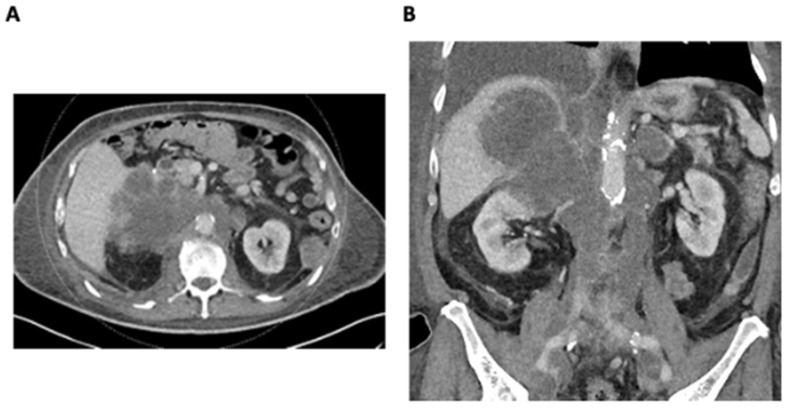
(A) Axial CT demonstrating a large infiltrative right adrenal metastasis and retroperitoneal lymphadenopathy. (B) Coronal CT demonstrating a large infiltrative right adrenal metastasis and retroperitoneal lymphadenopathy resulting in the compression and thrombosis of the IVC and iliac veins.
